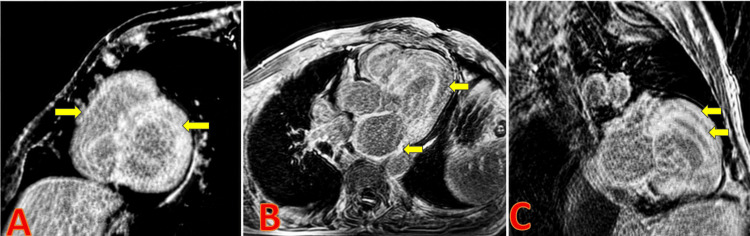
CMR imaging findings consistent with CA LGE CMR images demonstrating the characteristic diffuse subendocardial enhancement of ATTR-CM. (A) Short-axis view demonstrating diffuse circumferential subendocardial late gadolinium enhancement involving both the left and right ventricles (yellow arrows), consistent with biventricular amyloid infiltration. (B) Three-chamber (LVOT) view demonstrating diffuse subendocardial late gadolinium enhancement involving the left ventricle and left atrium (yellow arrows). (C) Long-axis view demonstrating diffuse circumferential subendocardial late gadolinium enhancement (yellow arrows), highly suggestive of CA. LGE: late gadolinium enhancement, LVOT: left ventricular outflow tract, CMR: cardiac magnetic resonance imaging, ATTR-CM: transthyretin cardiac amyloidosis, CA: cardiac amyloidosis
